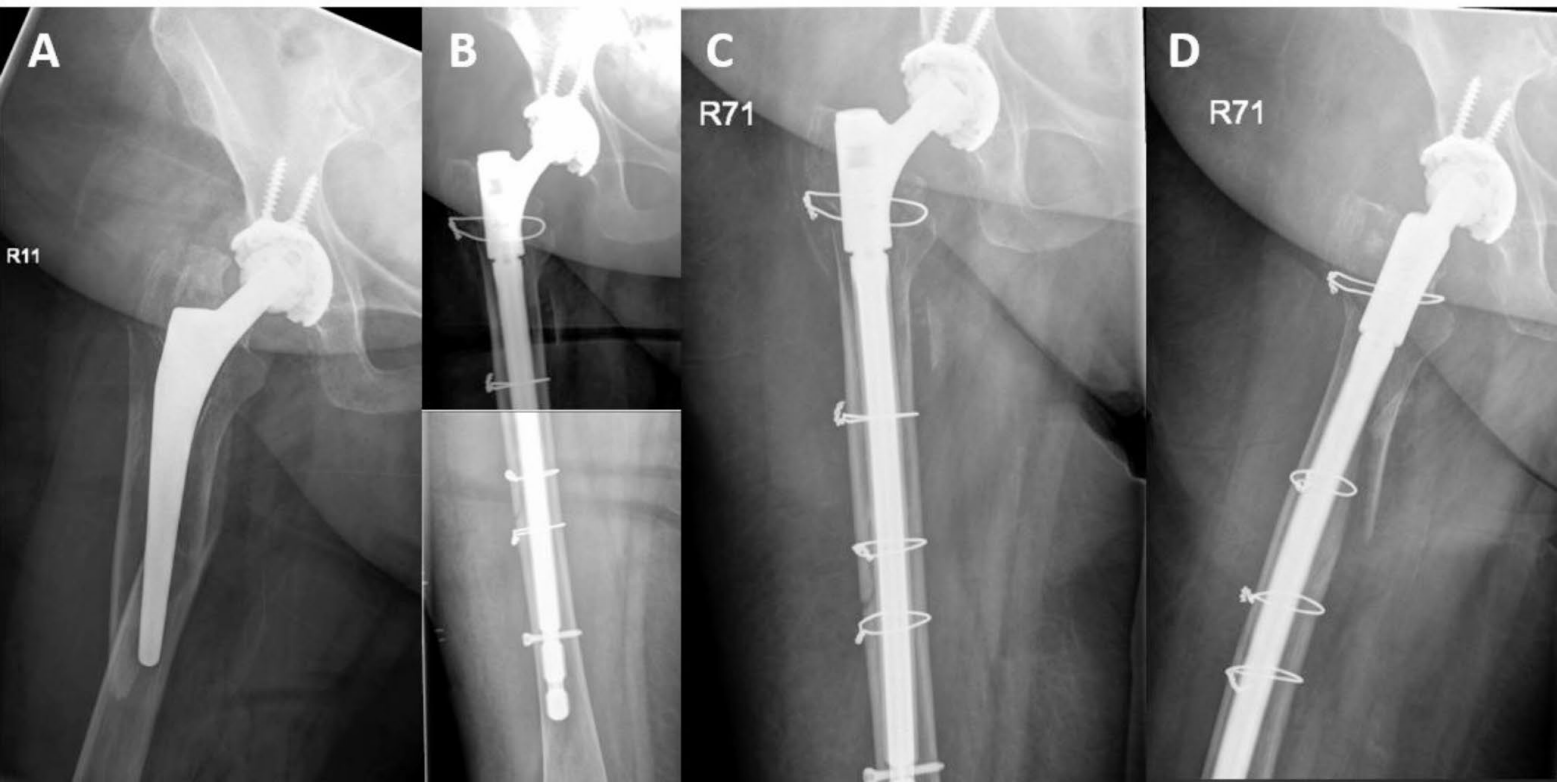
88 years-old female patient with a Vancouver B3 periprosthetic femur fracture who was operated during on-call hours. A) AP radiograph of the right hip after fall from standing height. B) AP radiograph at postoperative day 5 after revision arthroplasty. C) + D) AP and axial radiograph 6 weeks postoperatively showing stable conditions
